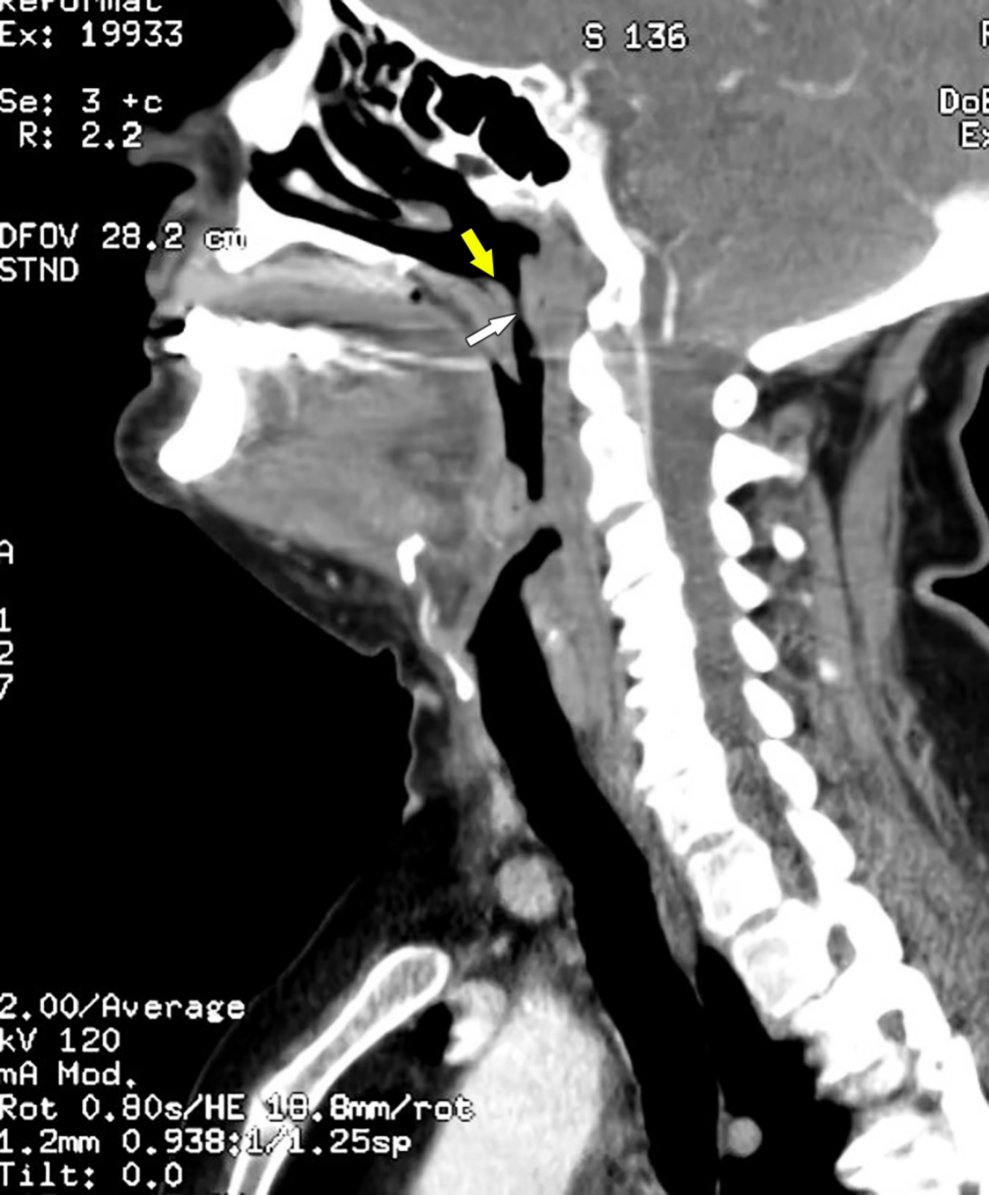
Sagittal view of CT soft tissue neck showing the enlarged lingual tonsils (yellow arrow) partially obstructing the airway (white arrow).
